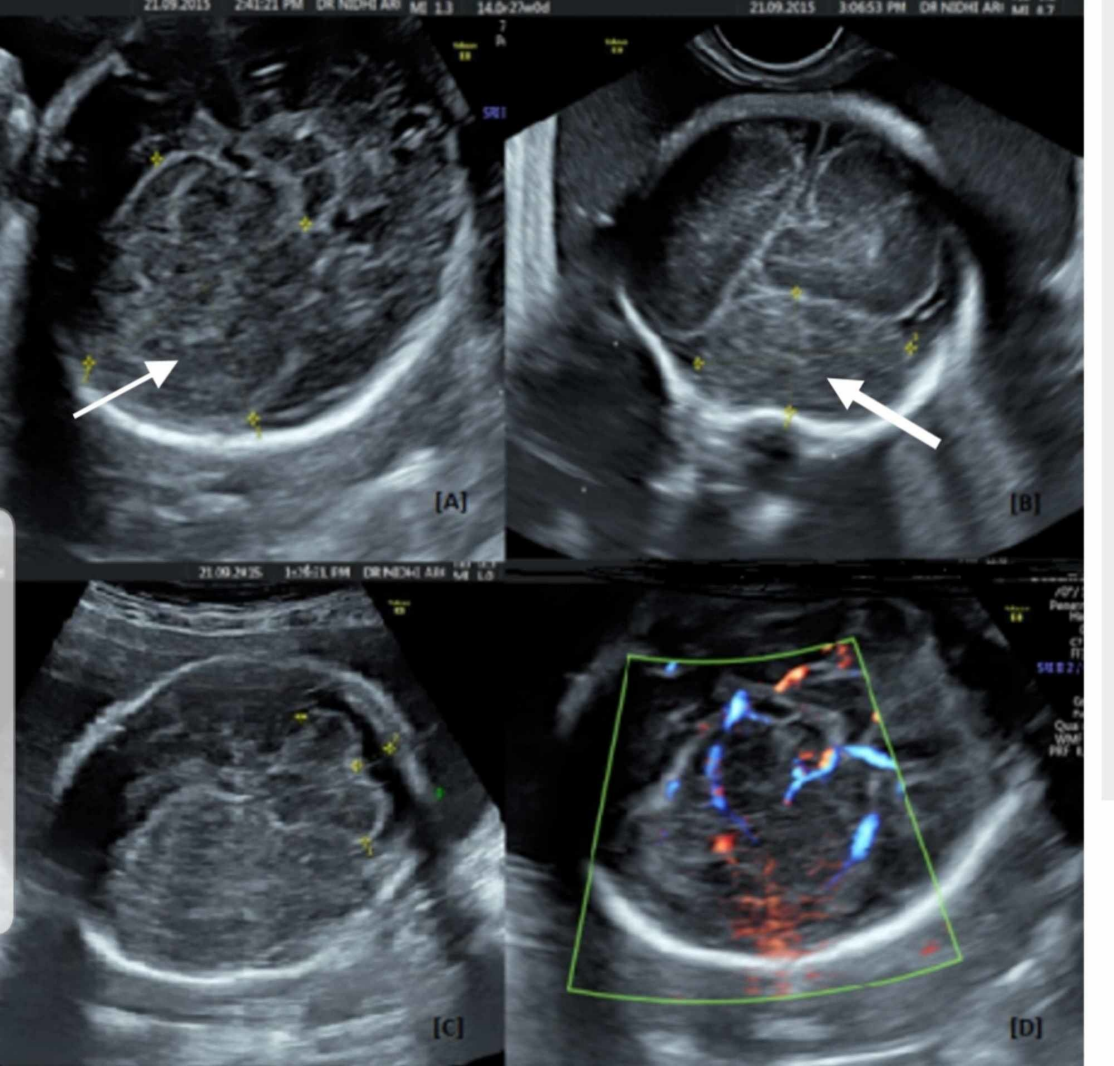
Ultrasound images of the intracranial mass. (A) 2D image showing homogenous mass in right frontal lobe in transthalamic plane (white arrow). (B) Coronal plane (thick white arrow). (C) Normal posterior fossa. (D) Color Doppler showing feeding vessels into the mass.
